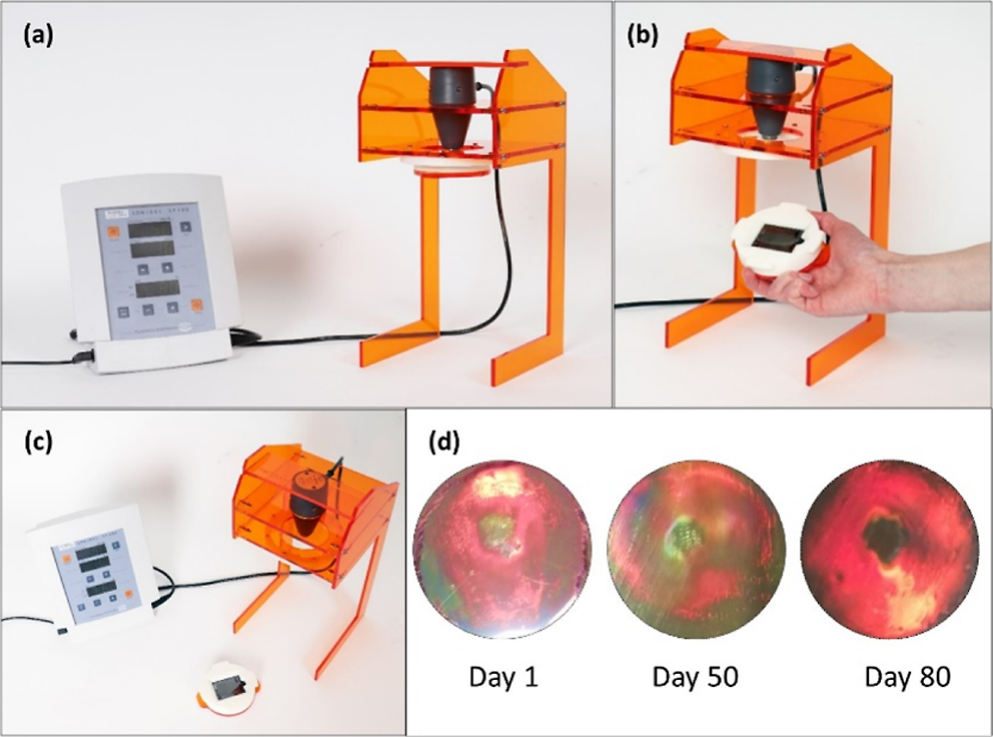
(a–c) Photographs of the prototype ultrasound testing
